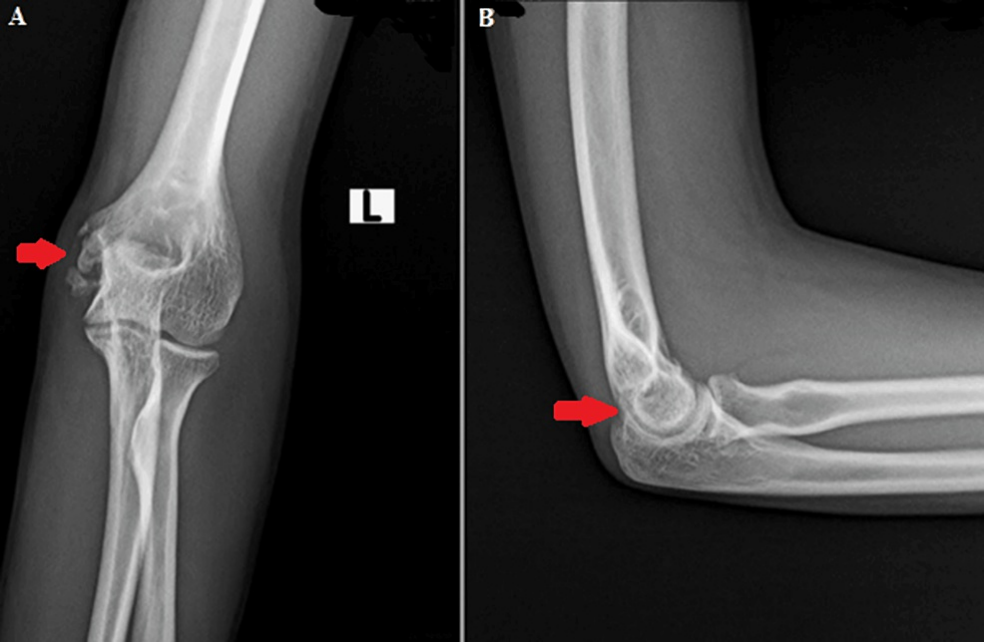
X-ray of the left elbow joint at one year of follow-up showing no signs of disease recurrence A: anteroposterior view; B: lateral view
